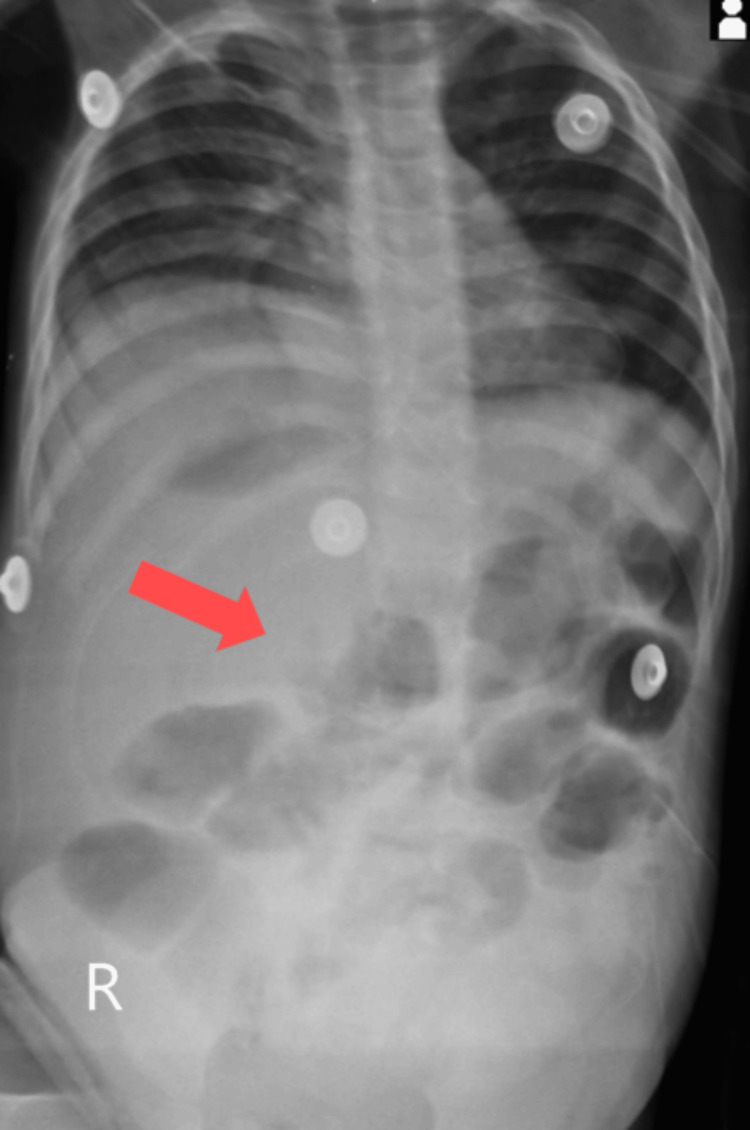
Abdominal X-ray showing distended bowel loops indicative of obstruction (red arrow).
